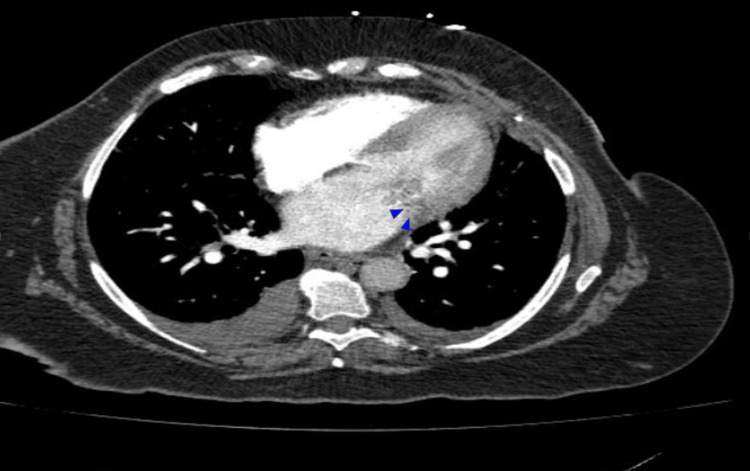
CTA pulmonary showing soft tissue density thickening along the left side of the mitral valve region (blue arrowheads) with some small internal calcifications. There was no pericardial fluid observed.
